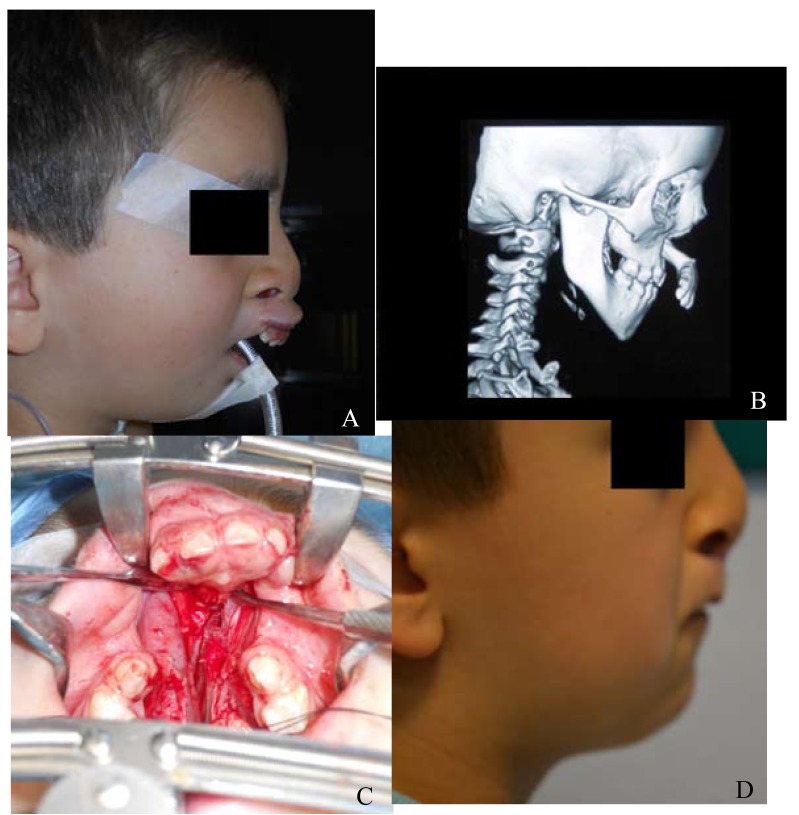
(case 1): A 4-year-old male with CBCL with protruding premaxilla and palate cleft. (A) Preoperative anterior and lateral view. (B) CT scan showing the extreme protrusion of the premaxilla with a vertical excess ; Notice the length of the vomerine bone needed to be excised on CT scan. (C) Occlusal intra-oral view, showing the unrestrained anterior nasal septal and vomero-premaxillary suture after wedge ostectomy of the vomer. (D) Postoperative results on lateral view (18 months postoperatively).
